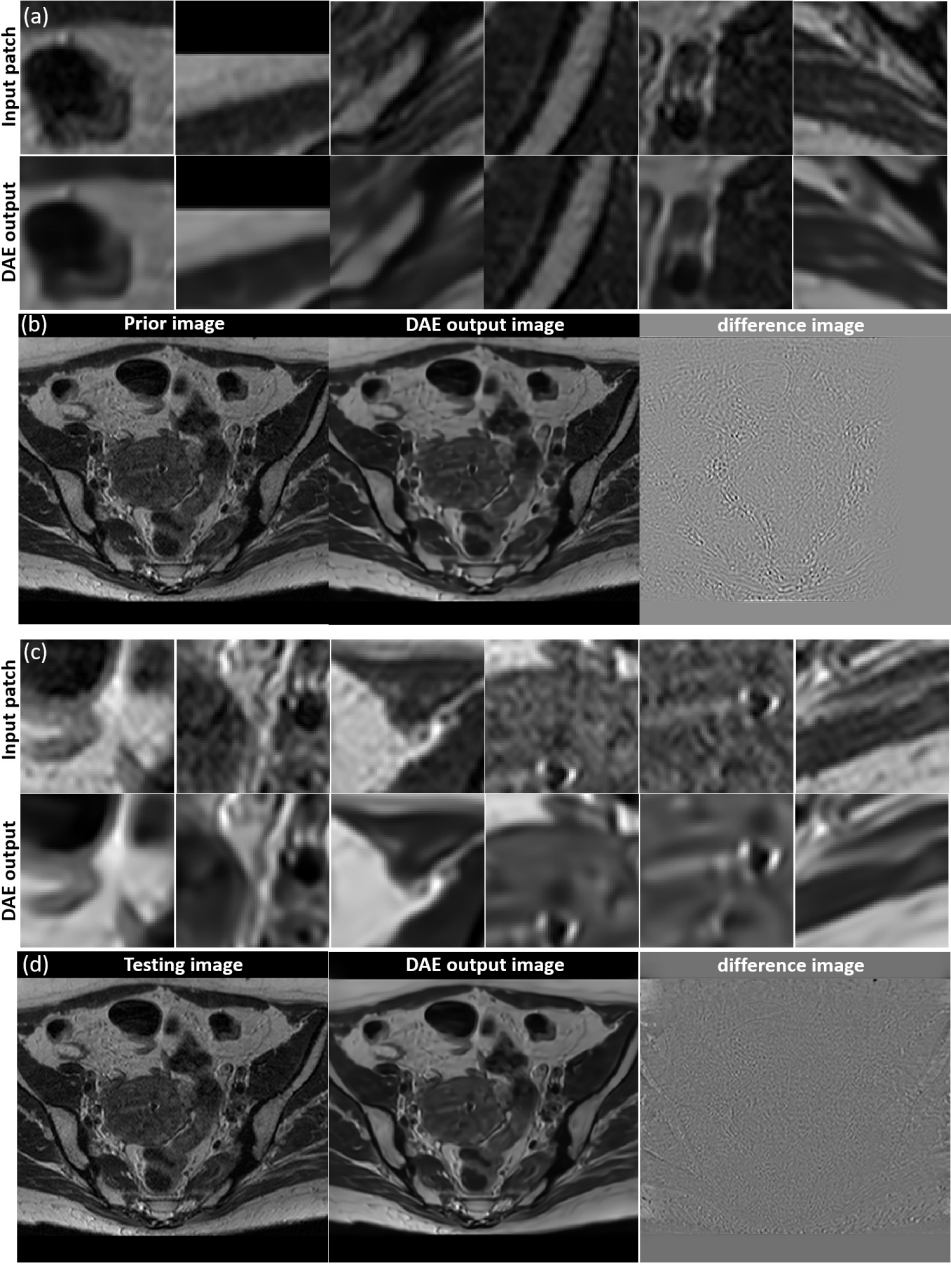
(A) Examples of input patches from the prior MR image and their corresponding outputs from the DAE. (B) The prior image, image formed by the DAE output patches, and their difference. (C) Examples of input patches from the test MR image and their corresponding outputs from the DAE. (D) The test MR image, the image assembled by patches from the DAE output, and their difference. All the MR patches and images are displayed in a window of [0, 1], while the difference images are displayed in a window of [-1,1].
